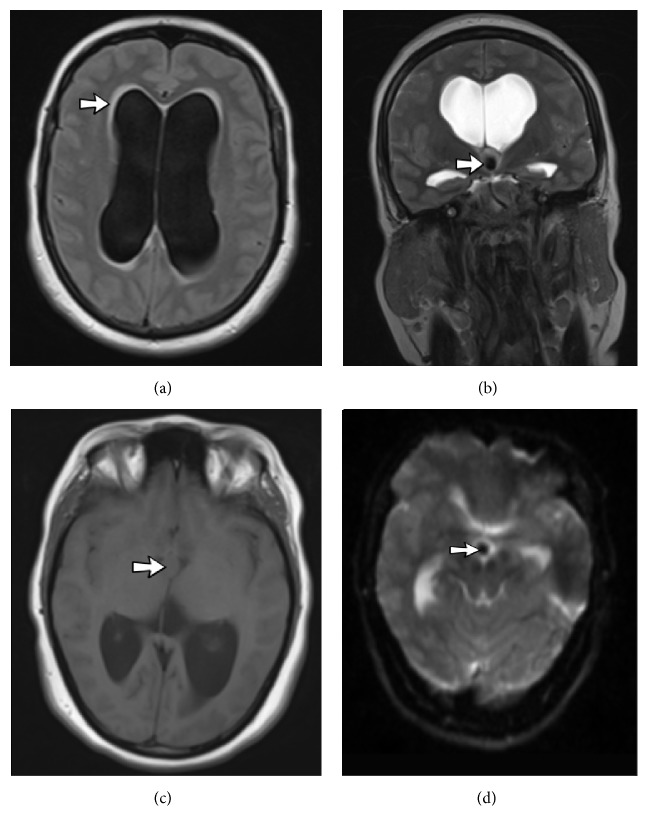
Brain MRI. Axial FLAIR image (a) shows enlargement of lateral ventricles. The hyperintense rim around the ventricles represents interstitial edema secondary to acute hydrocephaly. Coronal T2-weighted image (b) reveals a round hypointense lesion at the location of interventricular foramen of Monro (arrow in (b)). The lesion (arrow in (c)) is isointense in the axial T1-weighted image (c). The size of the lesion is exaggerated in the b0 sequence of DWI image (d); a finding that suggests hemorrhage (arrow in (d)).
